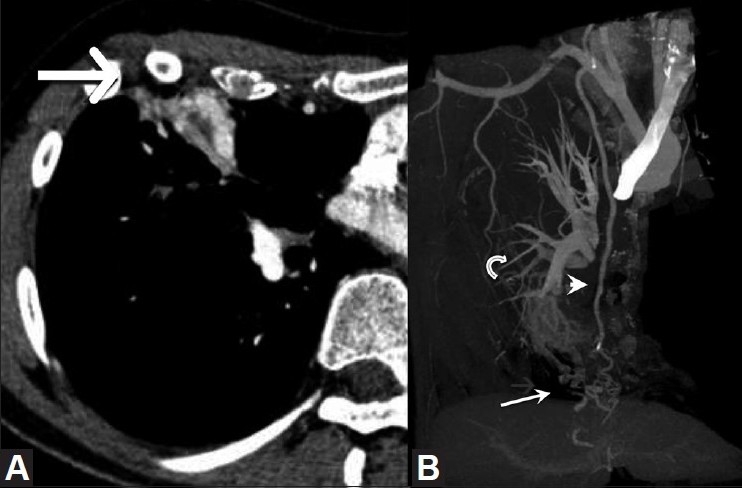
(A, B): Contrast-enhanced CT scan of the chest (A) demonstrates hypervascularity involving the consolidated right middle lobe (arrow). Bone-subtracted, maximum-intensity projection (B) shows a complex right middle lobe vascular malformation (arrow) supplied by the right internal mammary artery (arrowhead) with drainage into the right pulmonary artery (curved arrow)
